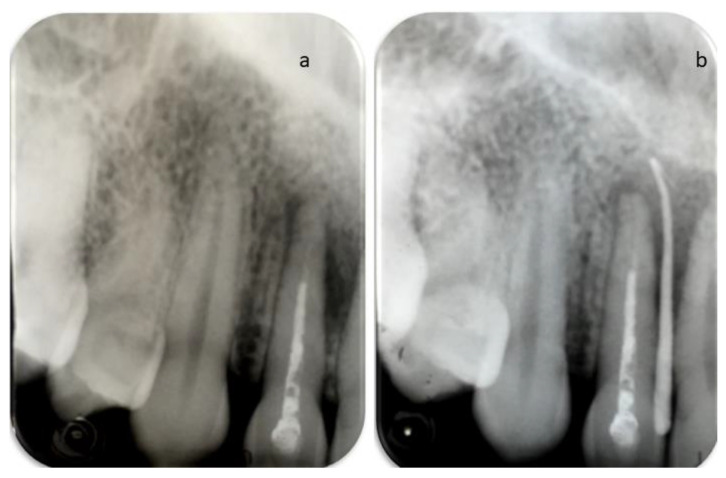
(a) Periapical radiograph depicting the presence of a previous root canal treatment on tooth 1.2 and a periapical radiolucency. (b) Periapical radiograph performed with insertion of a gutta-percha cone within the stoma to trace the sinus tract.
